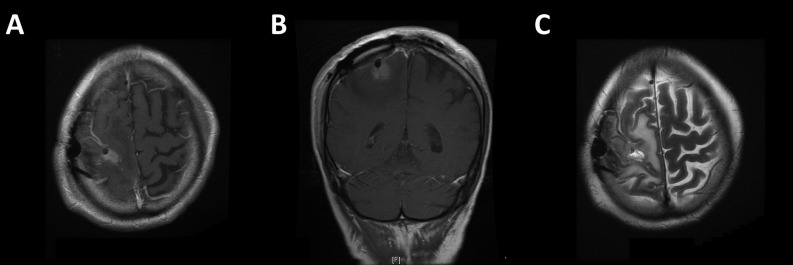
Postoperative MR images confirming gross total resection. A/B: Post-gadolinium T1-weighted images showing some hyperintense material in the cavity that does not enhance compared to pre-contrast sequence; C: T2-weighted image
